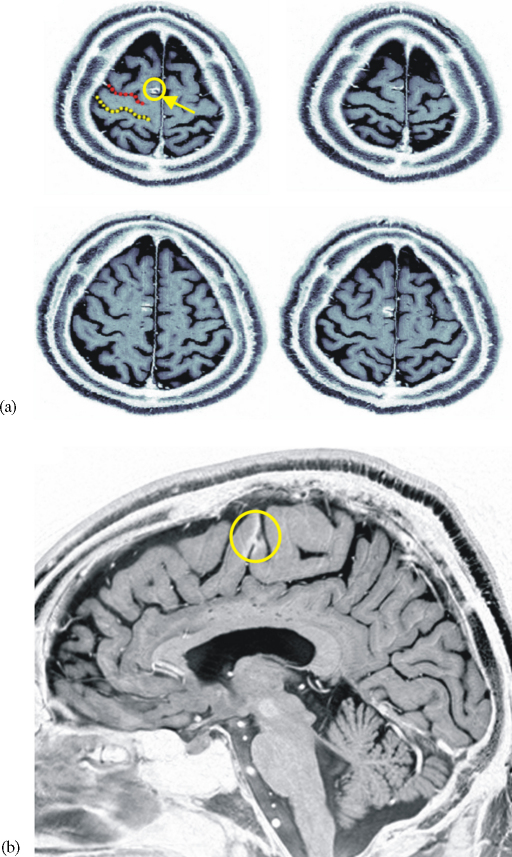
High-resolution structural MRI images presented with the grey scale inverted showing four axial slices (a) and a saggital slice (b) acquired on a 4.7 and 3 T scanner, respectively. The extent of venous infarction can be seen by the small area of signal change in (i) the left hemisphere on the axial slices and (ii) the dorso-medial area on the saggital image. On the axial slices, the precentral sulcus (red dots) and central sulcus (yellow dots) are marked on one slice, together with the paracentral sulcus (green arrow)—the anatomical landmark of the human SEF.
